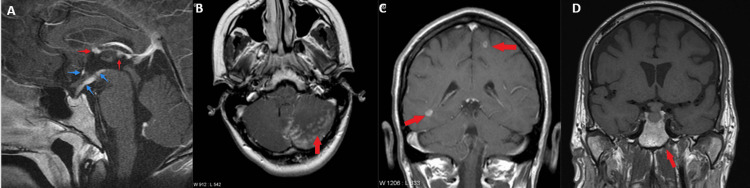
MRI showing the pituitary stalk (A), leptomeninges (B), brain parenchymal (C), and pituitary gland (D) revealing infiltrative lesions of neurosarcoidosis in the brain
